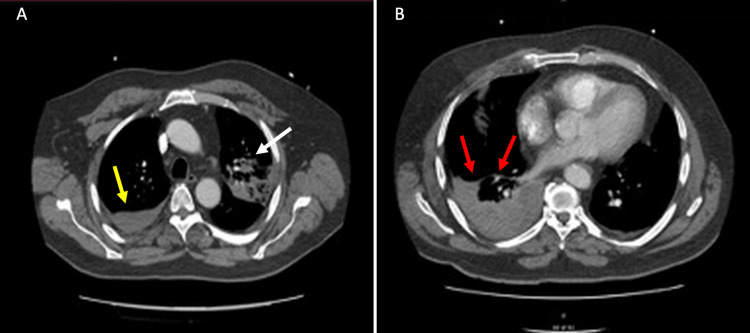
Computed tomography scan of the chest (A) Consolidation of left upper lobe (white arrow) with pleural effusion on the right side of the lung (yellow arrow), and (B) mild pleural effusion on the right lung field (red arrow)
